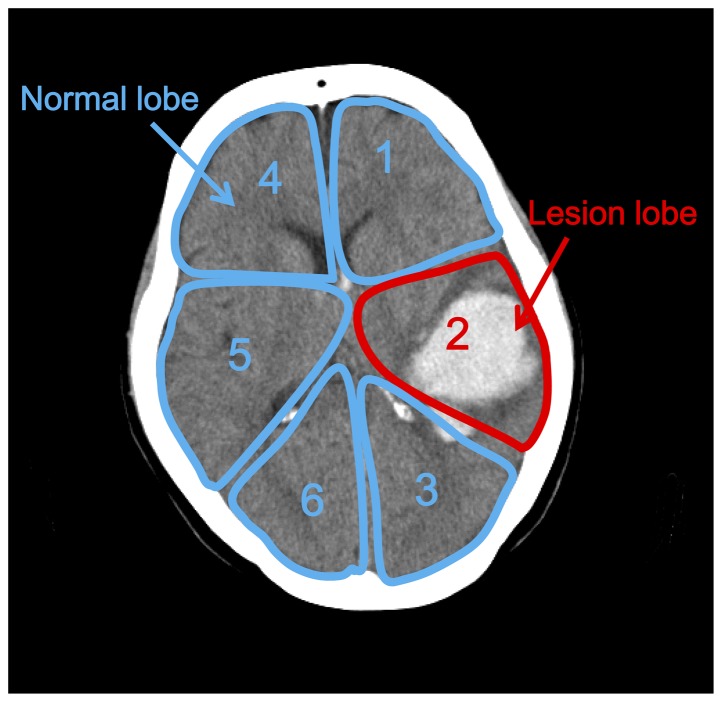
The definition of Regions of Inerest (ROIs). The brain is segmented into six lobes according to anatomy, including left frontal lobe, right frontal lobe, left temporal lobe, right temporal lobe, left occipital lobe and right occipital lobe. The six lobes are then classified into normal lobes and lesion lobe according to corresponding CT images for subsequent impedance observation.
